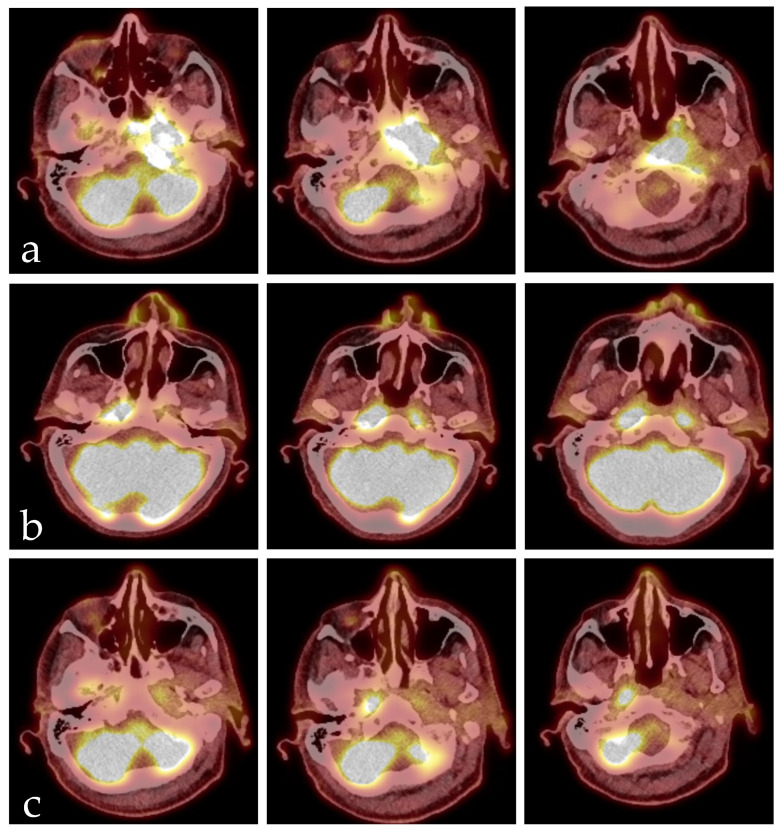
Exemplary representation of FDG-PET-CT scans the patient received from March until October 2025; CT scans are merged with PET scans. (a) Initial scan from March 2025 with an intense accumulation in the nasopharynx on the left side and broad-based infiltration of the skull base/clivus. (b) Second scan after six weeks of systemic antibiotic therapy with meropenem, showing a significant reduction in the size of the lesion in the left nasopharynx. However, a new accumulation has appeared in the right nasopharynx. (c) Scan two months after discontinuation of antibiotic therapy, showing only discrete residual storage visible.
